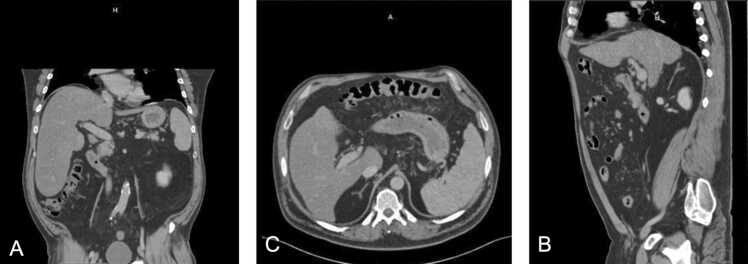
Repeat abdominal CT scan with contrast on follow-up 3 month later (portal vein thrombosis has resolved) a) coronal, b) transverse and c) sagittal.
